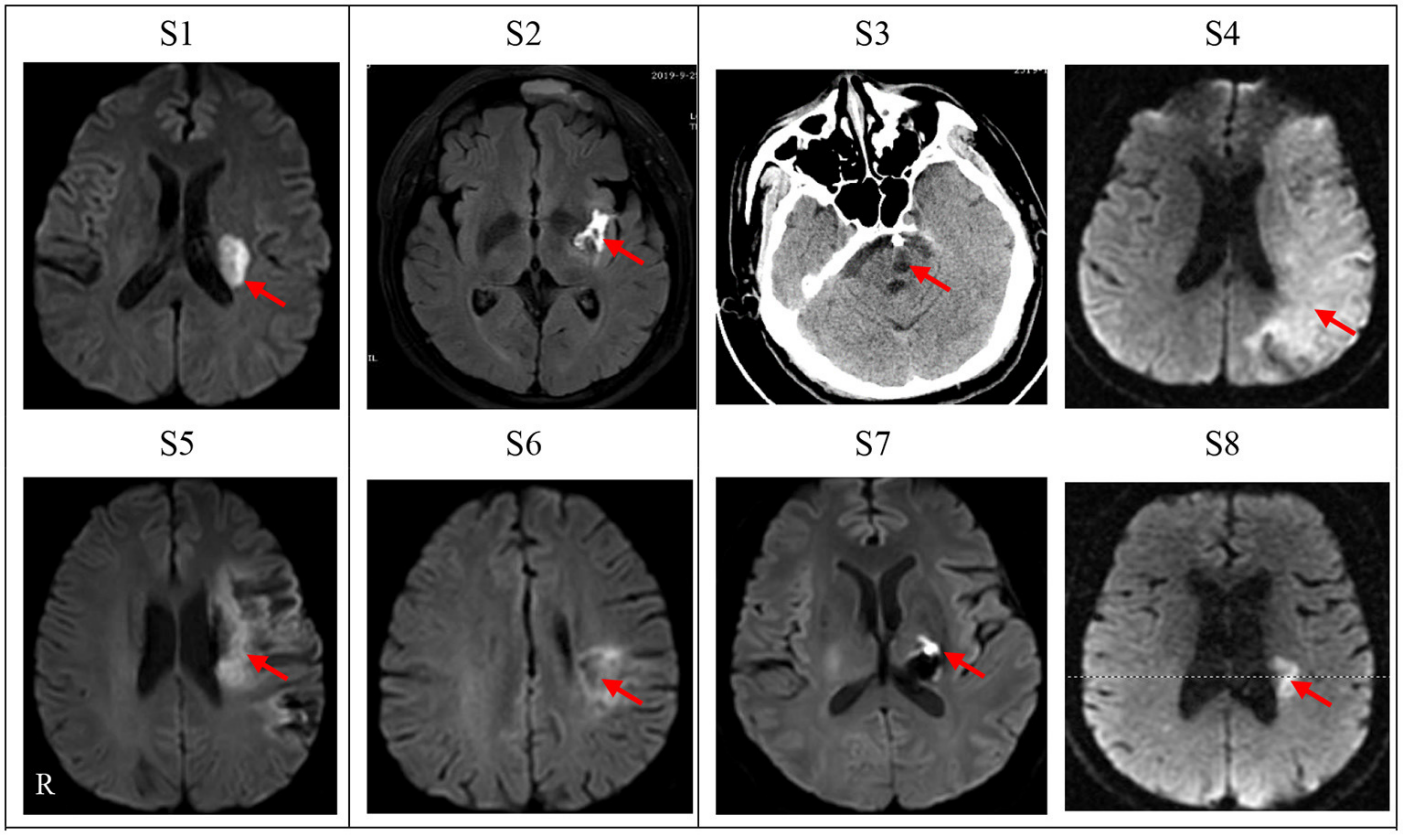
Diffusion weighted-MRI Images or CT images for cases *n* = 8). Lesion location of each subject was shown as diffusion weighted-MRI scan images except one subject (S3) with CT scan who had an intracranial metal clip. S1–S8 denotes the eight patients in this study. Left side of figures represents right side of the subjects. R, Right.
